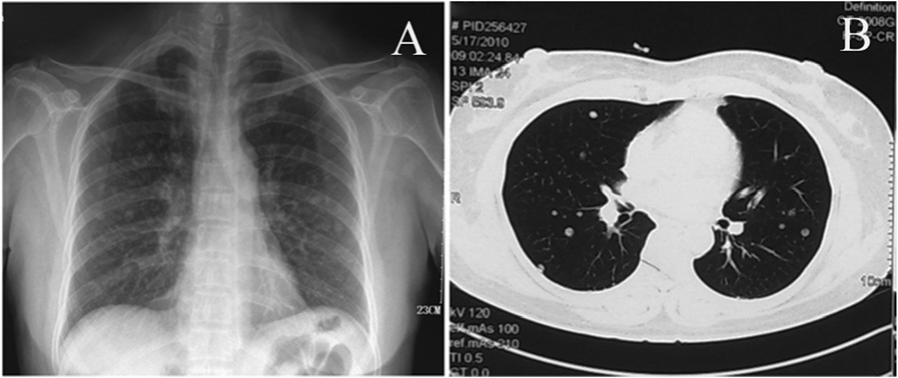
X-ray and CT images for Case 3. Posteroanterior chest X-ray reveals bilateral diffuse nodular opacities (A). CT shows multiple nodules in both lungs (B).
